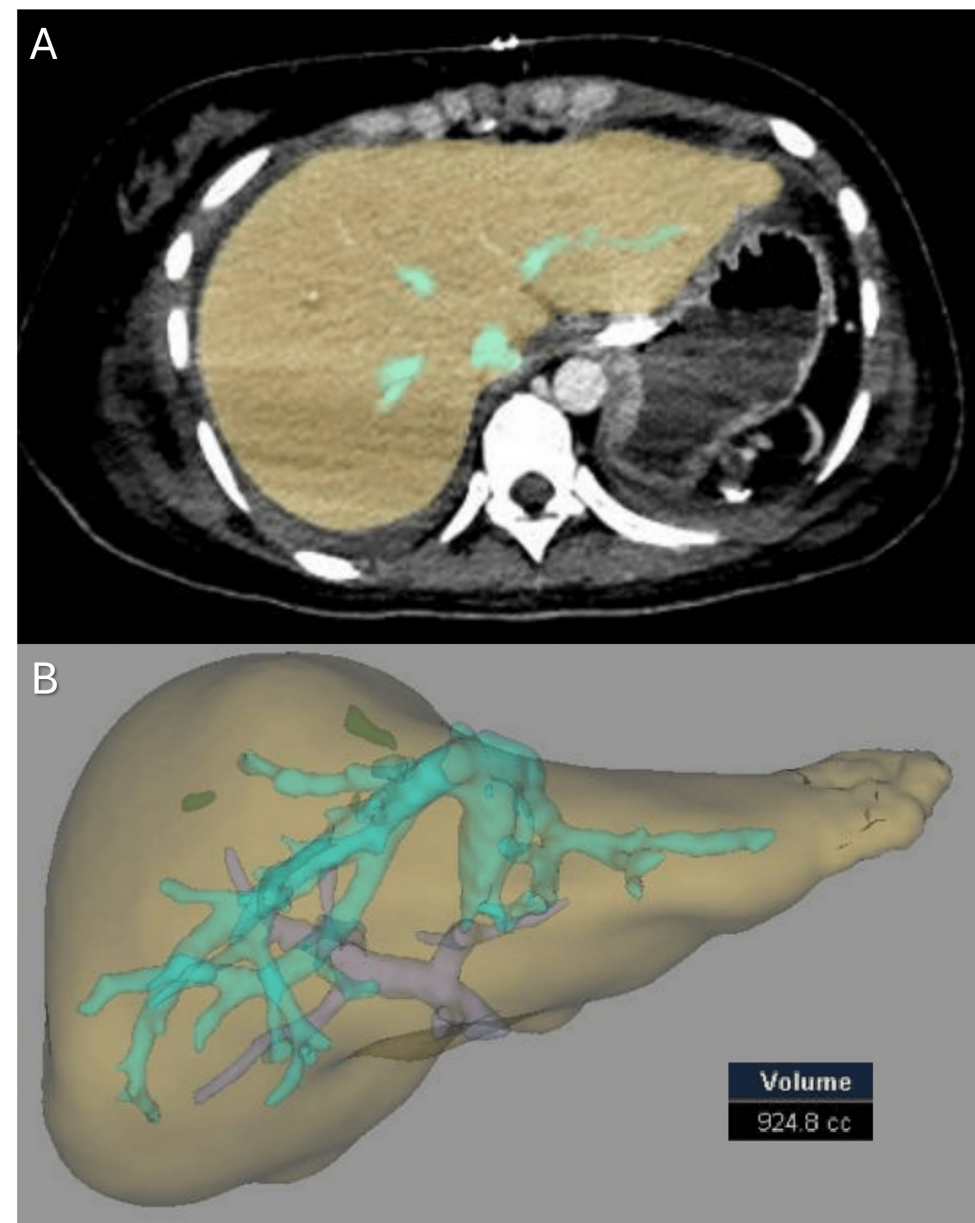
Unenhanced abdominal computed tomography on postoperative day 6 showing (A) no intra-abdominal complications, the presence of an incisional hematoma, and (B) a liver graft volume of 924.8 cc.
